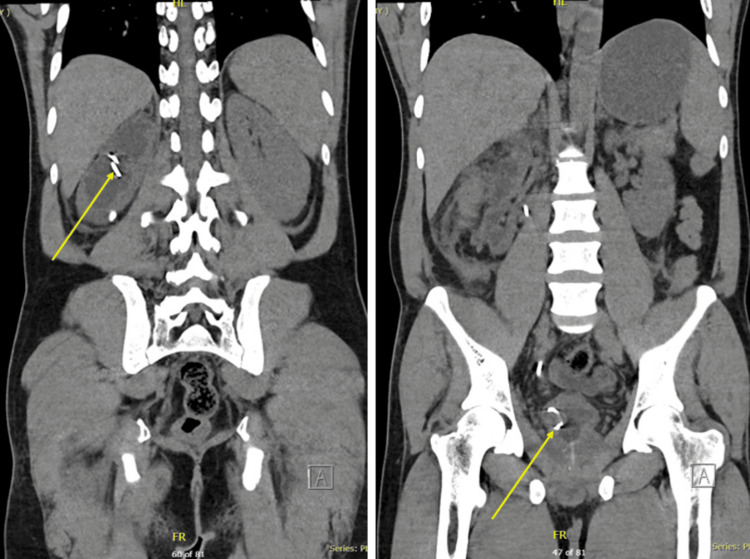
CT image demonstrating the in-situ ureteral stent (yellow arrow).
